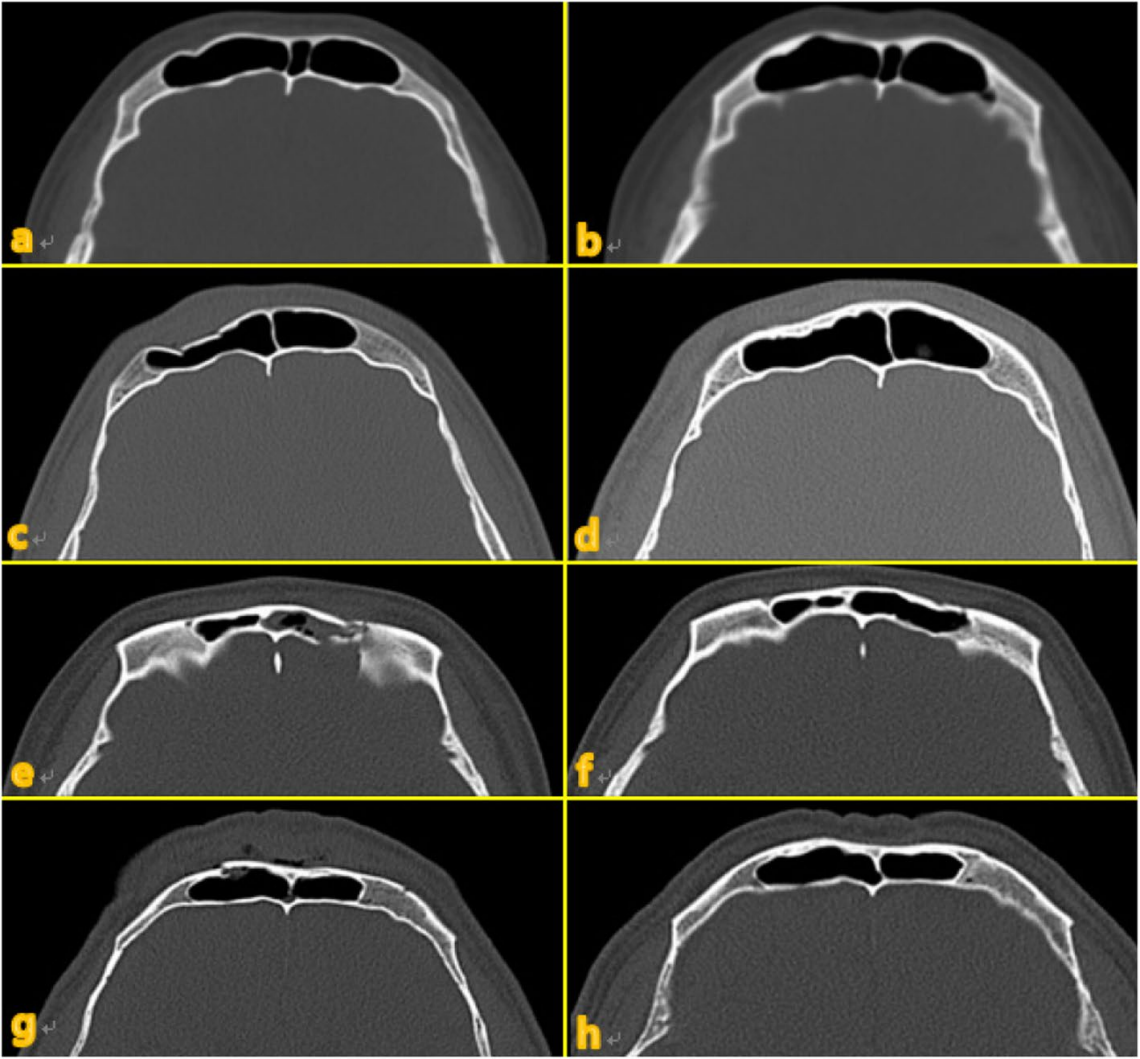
a, c, e, and g Computed tomographic imaging at the time of injury. b, d, f, and g Post-injury images at 9 years and 9 months, 6 years and 7 months, 1 year and 8 months, and 4 years and 11 months, respectively
